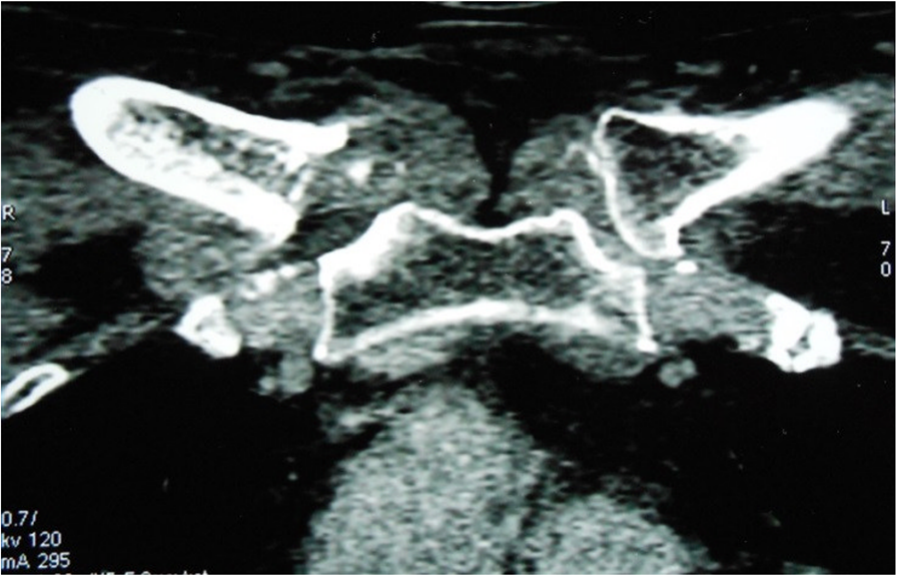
Computed tomography scans of the sternoclavicular joints demonstrating destruction of the medial extremities of the clavicles
